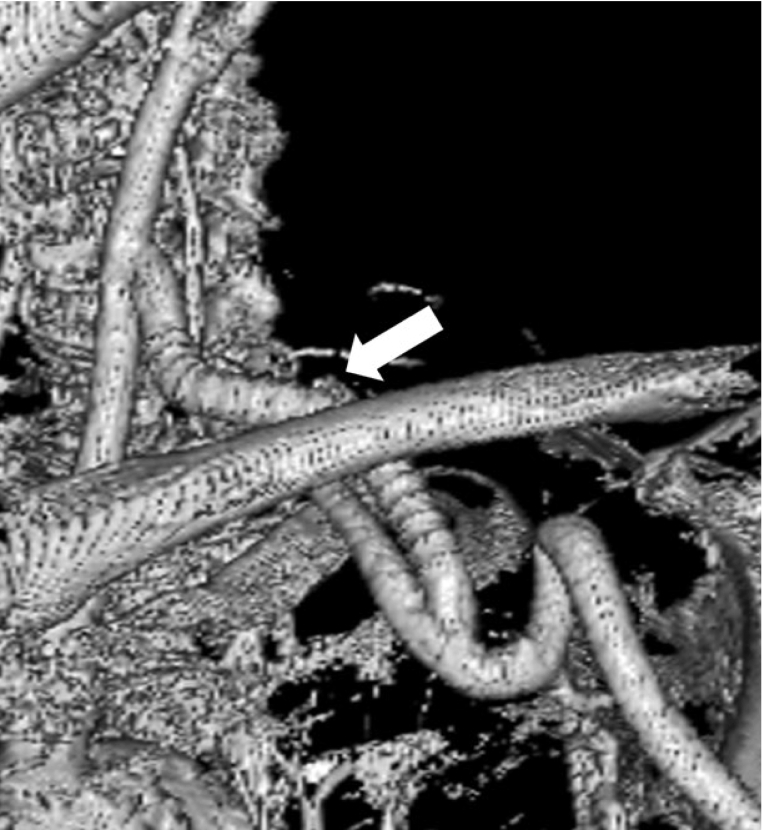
Three-dimensional computed tomography images 1 week post-operatively. The bypass graft is patent (white arrow).
